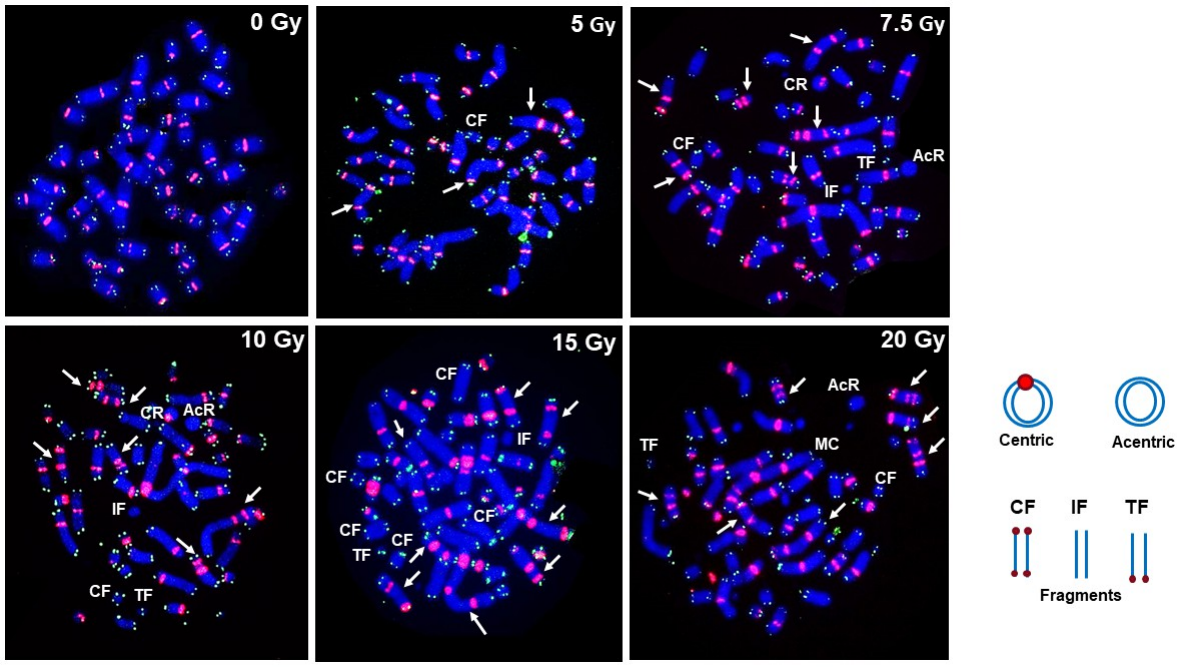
Representative pictures of calyculin A induced G2-PCCs prepared from mock and irradiated lymphocytes with different doses of x-rays. Dicentric and multicentric chromosomes are indicated by arrows. CF-Compound fragment, TF- Terminal fragment, IF-Interstitial fragment, MC-Multicentric chromosomes, AcR-Acentric ring and CR-Centric ring.
